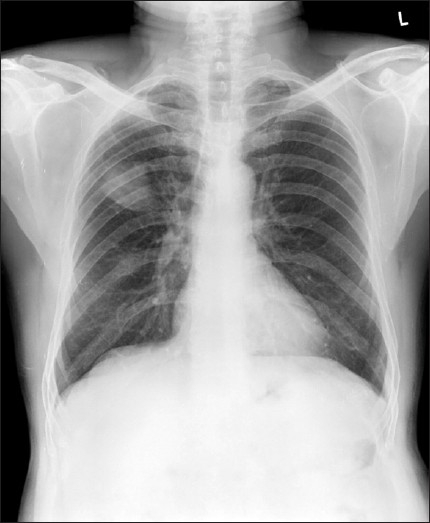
Chest radiograph showing a solitary pulmonary mass lesion in the right upper lobe
